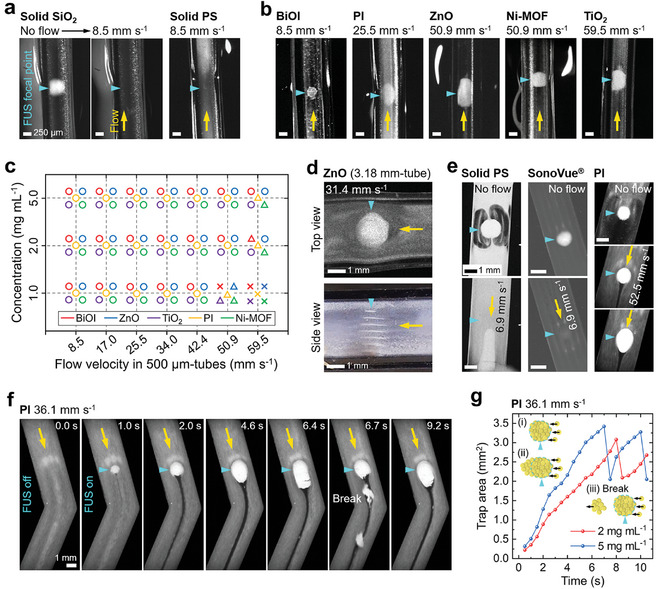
Observation of acoustic trapping under dynamic fluid flow. a, b) Top‐view of the solid SiO_2_ and PS MPs (a), and 5 HNS‐MPs (b) flowing inside the 500 µm‐diameter tubes while focused ultrasound (FUS) was activated. The cyan triangle marks the focal point of FUS and the yellow arrow indicates the direction of water flow. c) Summary of acoustic trapping performance of different HNS‐MPs at various concentrations and flow velocities. The performance test was conducted by using the 500 µm‐diameter tubes shown in Figure 2b. “°” indicates successful trapping in established flow, “△” corresponds to trapping only when a trap is formed before flow, and “×” implies no trapping. d) Top‐ and side‐views of the ZnO MPs trapped inside the 3.18 mm‐diameter tube under water flow. e) Photographs showing the trap formation of solid PS MPs, SonoVue microbubbles, and PI MPs under static flow conditions, and their changes with water flow. f) Time‐dependent photographs showing the formation of a PI MPs trap under an established water flow and its changes inside the microfluidic channel. g) Changes in the area of PI MPs trap over time with different concentrations. The FUS was activated at 0 s.
